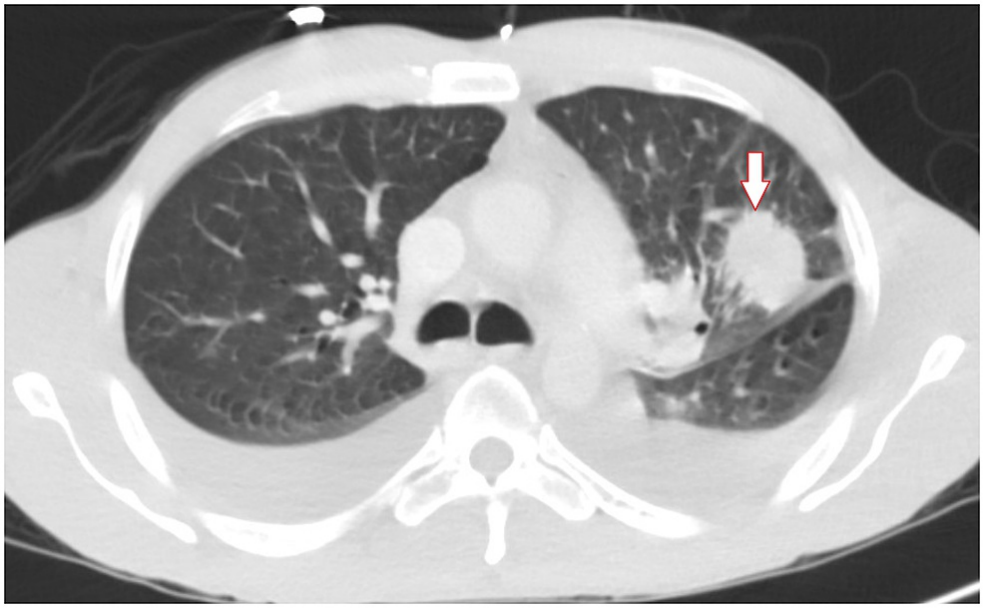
3.6 cm spiculated mass in the left upper lobe shown on CT scan of the lung (white arrow)
